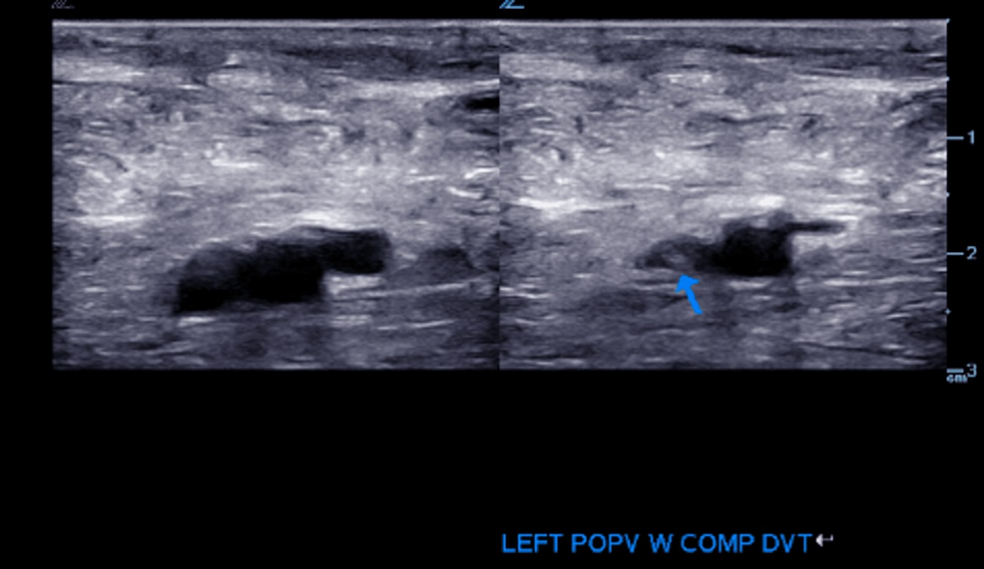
Venous duplex ultrasound: cross-sectional view: left popliteal vein with thrombus.
